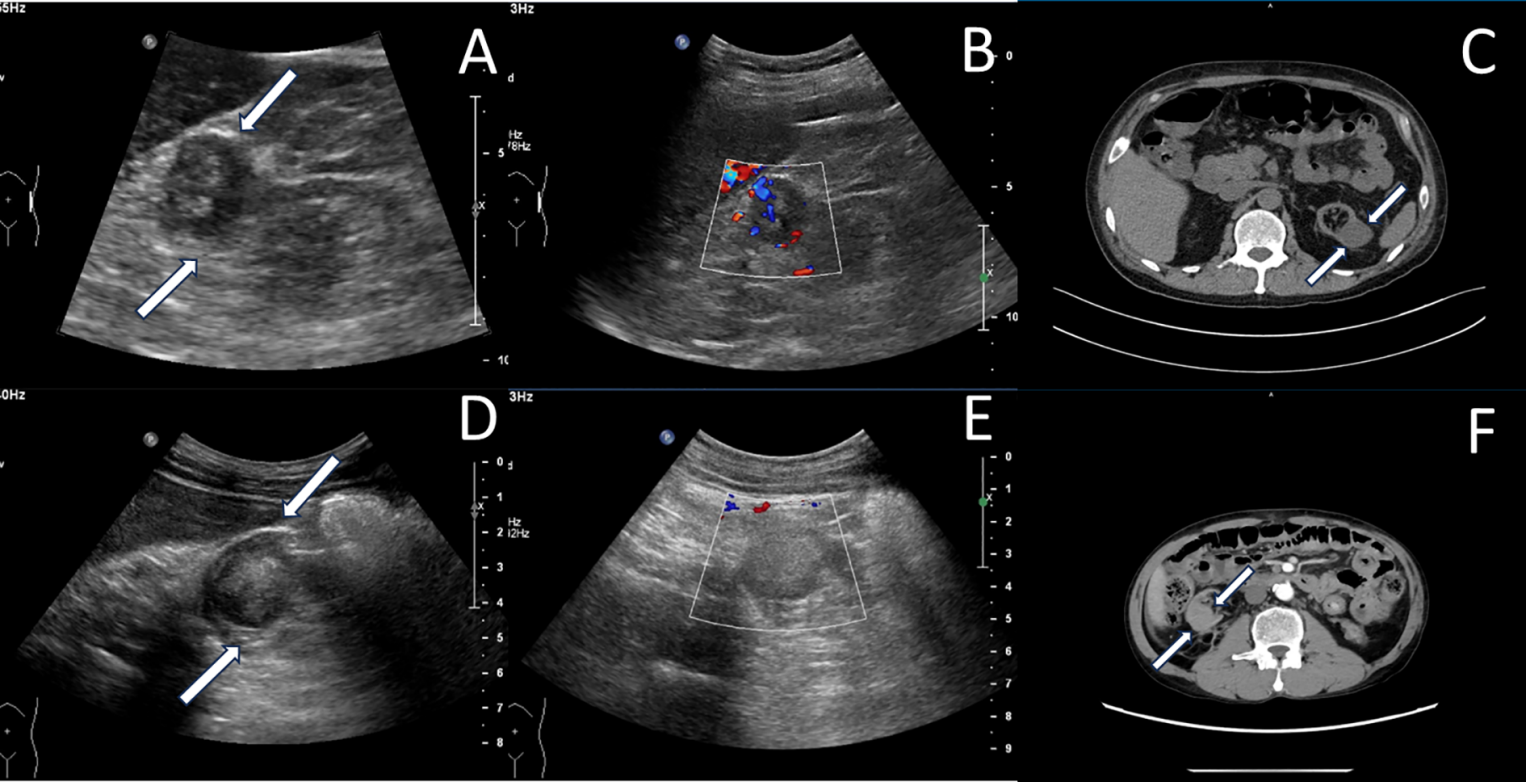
Native renal tumors in other cases. Case 2: (A) A hypoechoic mass(white arrow) with relatively clear boundaries was detected in the upper portion of the left native kidney. (B) Color Doppler shows intralesional vascularity (dot/linear signals). (C) Computed tomography showed a slightly low-density mass in the superior-lateral portion of the left kidney (white arrow). Case 3: (D) A hypoechoic mass(white arrow) was detected in the middle and lower portions of the right native kidney. (E) Color Doppler ultrasound showed absence of vascularity within the mass. (F) Enhanced computed tomography demonstrates heterogeneously enhancing mass in atrophic right kidney (white arrow).
